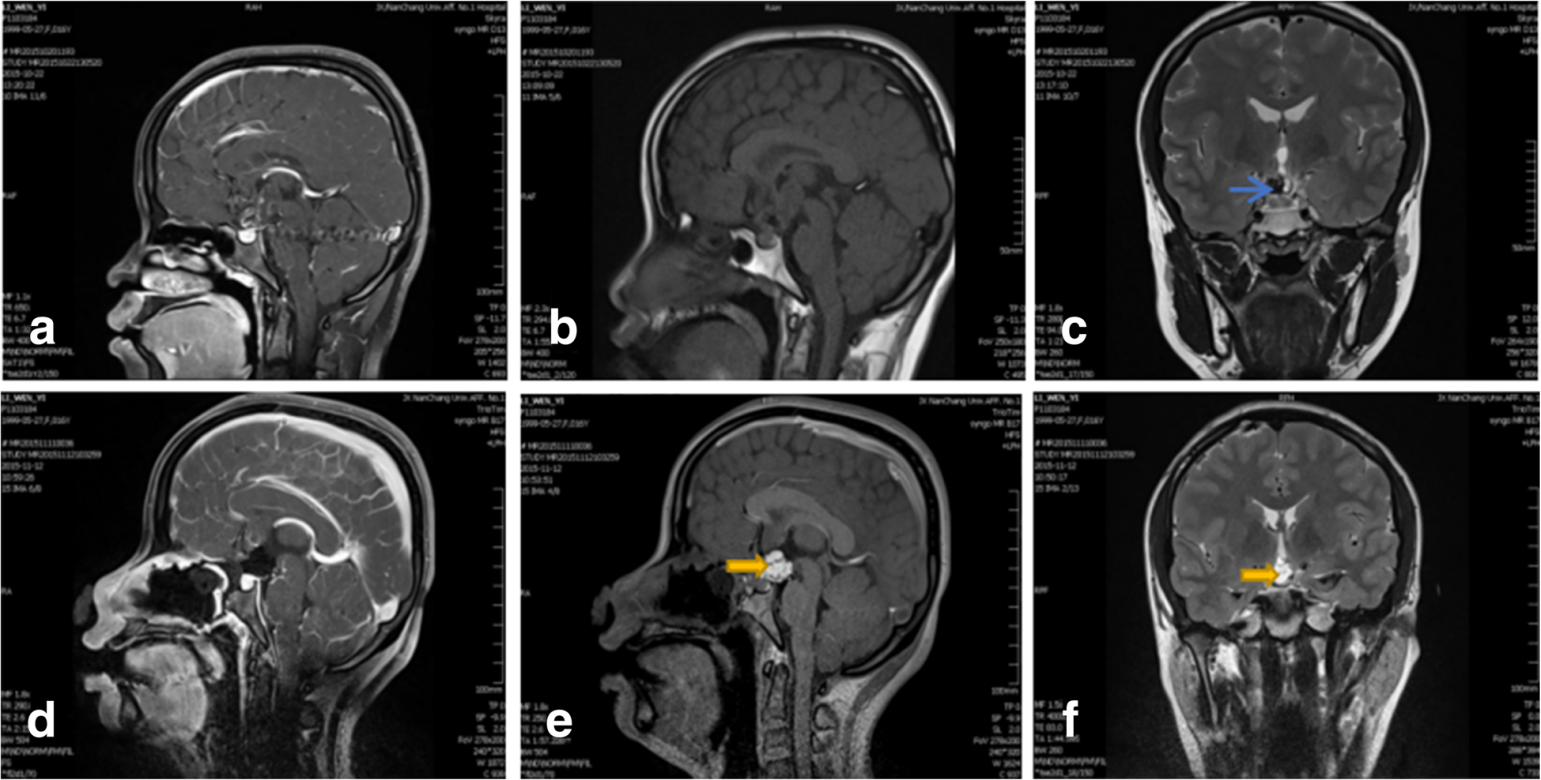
The pre- and post - operative MR images. a, b, c,: sagittal T1-weighted image with contrast enhancement and T1-weighted image (a, b) and coronal T2-weighted image (c) taken before endoscopic surgery showed a lesion in sellar area with calcification. d, e, f: the four images taken on postoperative day 2 showed the craniopharyngioma lesion was total resected. The green arrow showed calcification in tumor; the Yellow arrows showed auto-adipose tissue
